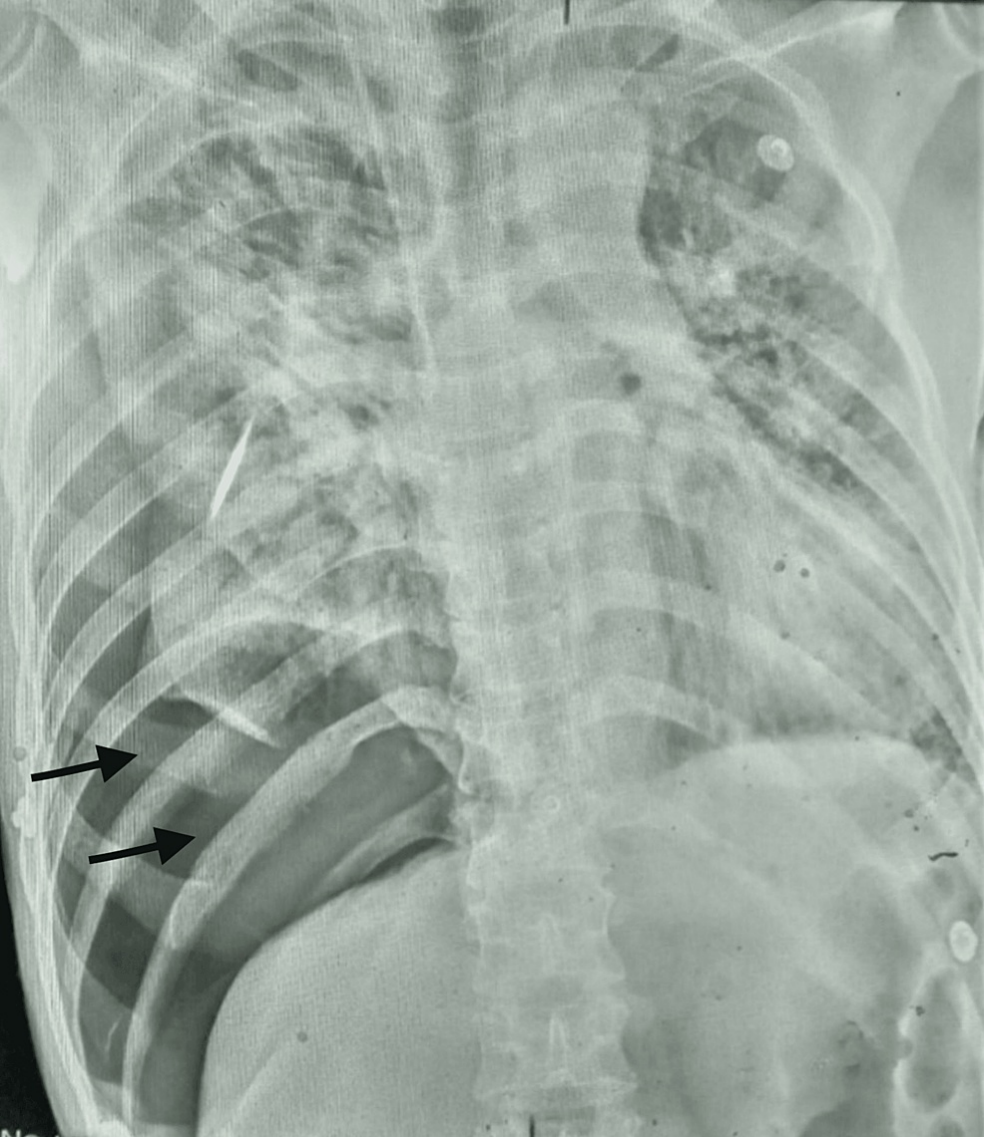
Initial chest X-ray showing right-sided pneumothorax (black arrows).
